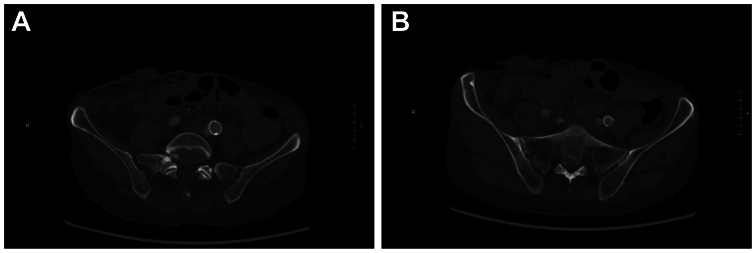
Computed tomography angiography (CTA) demonstrating an endoleak around the iliac aneurysm sac (A) and patent bilateral internal iliac arteries (IIAs; B).
